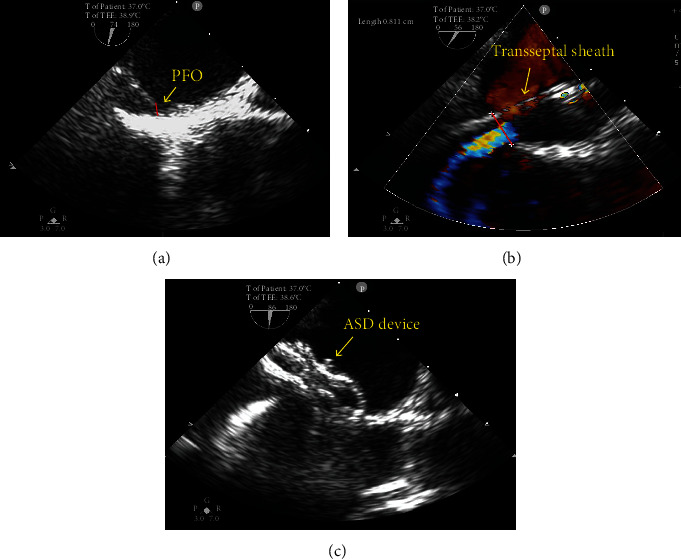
Implantation of the ASD device under TEE guidance. (a) The left height of the PFO after VM was 3 mm. (b) TEE displaying the 8 mm PFO-SD when the delivery sheath passed the PFO. (c) TEE displaying the proper shape and position of the 14 mm ASD device. ASD: atrial septal defect; SD: stretch diameter; PFO: patent foramen ovale; TEE: transesophageal echocardiography; VM: Valsalva maneuver.
